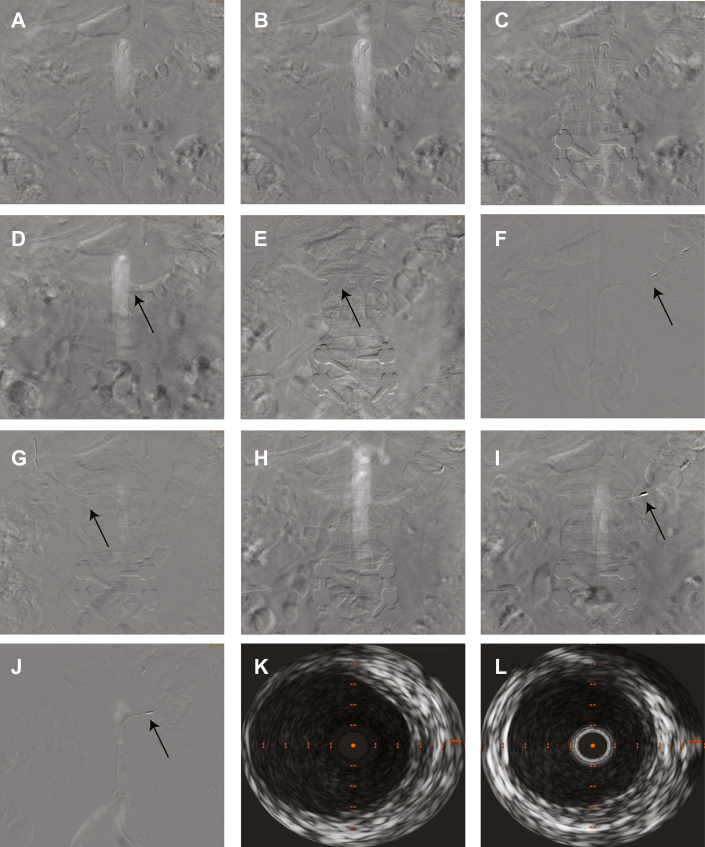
Aortograms, CO_2_ Angiography of the Renal Arteries, IVUS Imaging, and Pre- and Post-uRDN Imaging (A to C) Aortograms obtained using CO_2_ angiography revealing (A) the descending aorta and proximal sections of the bilateral renal arteries, (B) the descending aorta and proximal and mid sections of the bilateral renal arteries, and (C) the abdominal aorta and its bifurcation into the bilateral common iliac arteries. (D and E) Selective CO_2_ angiography of (D) the left renal artery and (E) the distal right renal artery showing distal bifurcation. (F to H) IVUS and post-uRDN imaging. IVUS images of (F) the left renal artery before uRDN therapy and (G) the right renal artery after uRDN therapy. (H) Aortogram obtained using CO_2_ angiography after uRDN therapy revealing the left renal artery with no aneurysmal changes or dissection. (I and J) Occlusion testing of the uRDN catheter at the (I) distal and (J) proximal left renal artery. (K and L) IVUS images of (K) the left renal artery before uRDN and (L) the right renal artery after uRDN. CO_2_ = carbon dioxide; IVUS = intravascular ultrasound; uRDN = ultrasound-guided renal denervation.
